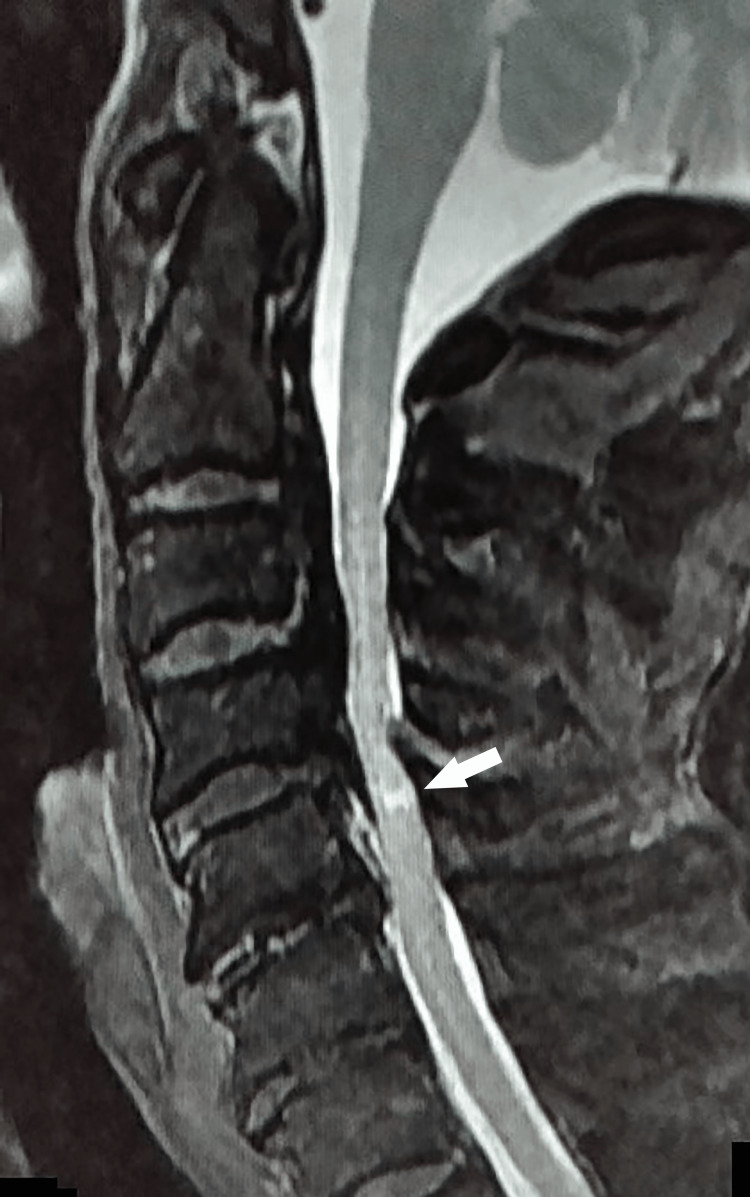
Preoperative T2-weighted cervical spine sagittal MRI Preoperative MRI revealed a severe spinal canal stenosis due to an ossified posterior longitudinal ligament at C3–C5, accompanied by intramedullary high signals in the T2-weight image (arrow).
